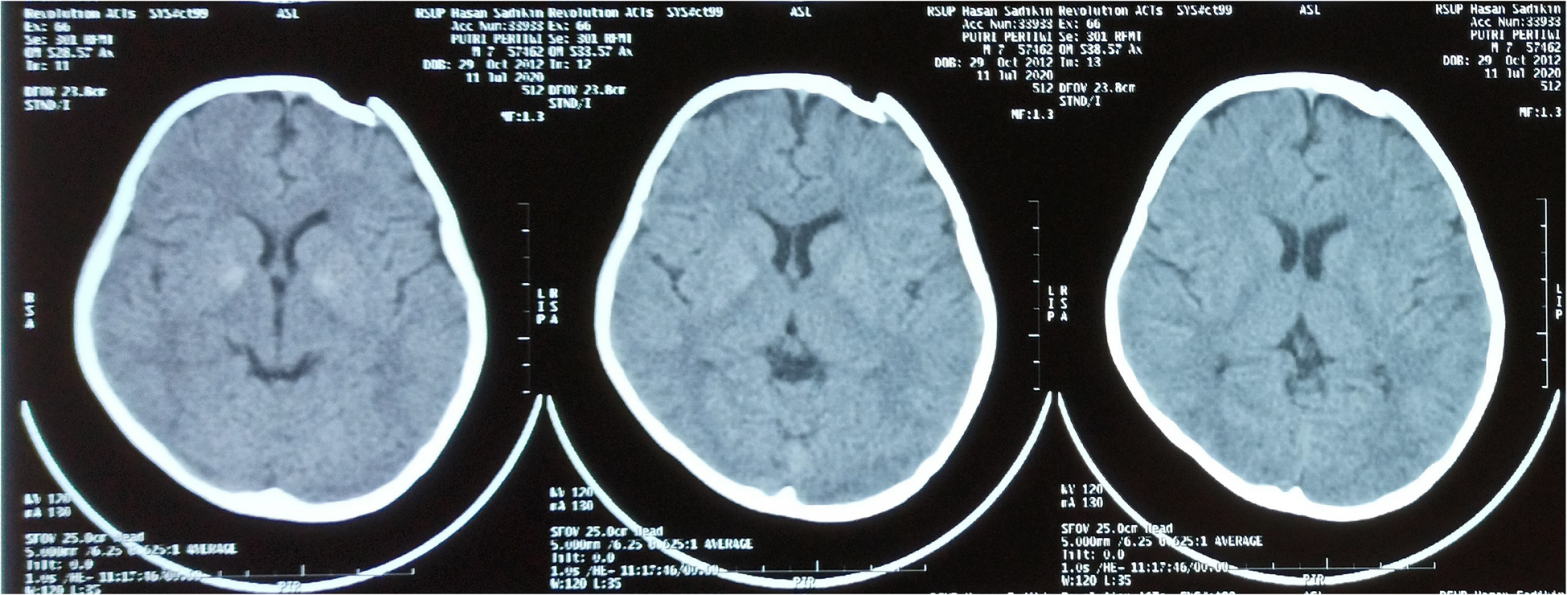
Contrast head CT scan which showed a depressed skull fracture of the left frontal bone
